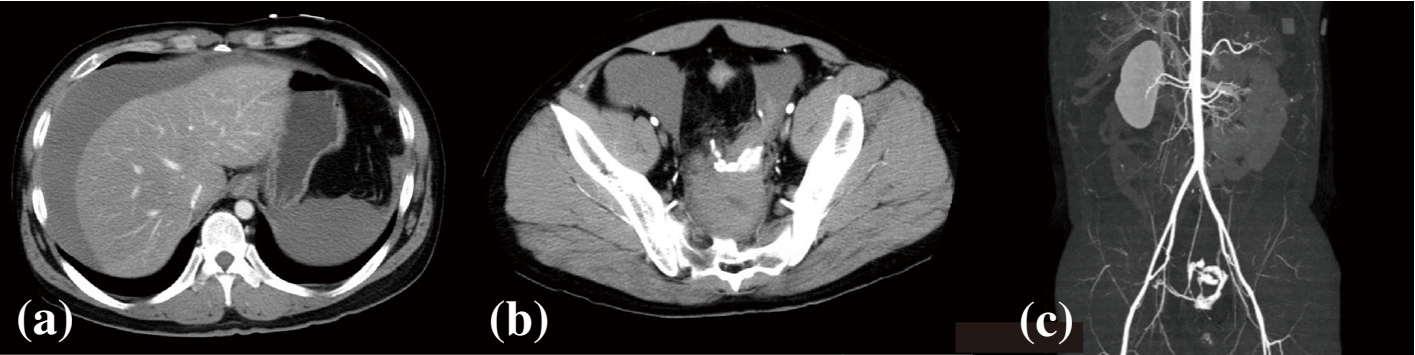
Contrast-enhanced abdominal computed tomography on admission. (a, b) Accumulation of hemorrhagic ascites and extravasation of radiopaque dye into the pelvic cavity. (c) A three-dimensional reconstruction of the computed tomographic image.
